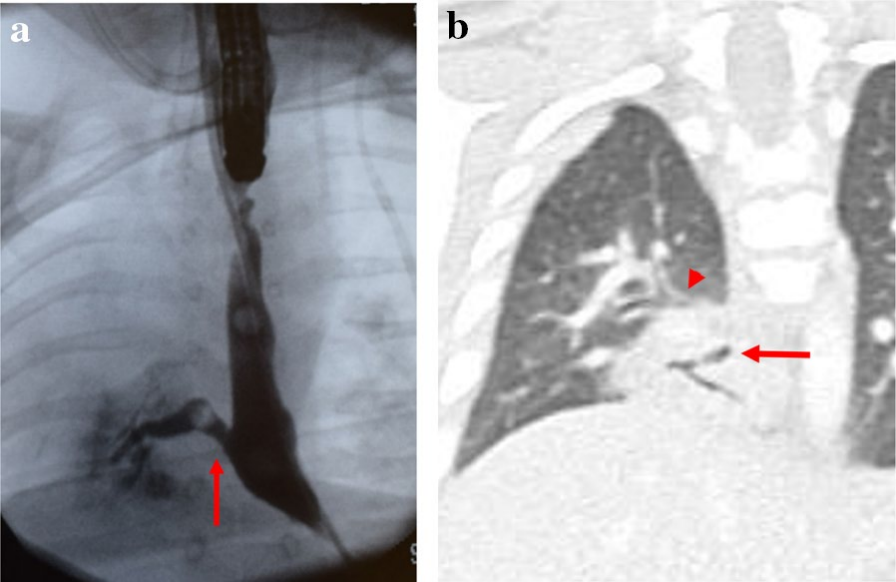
a An Esophagogram findings. The connection between the lower esophagus and right lower lung (arrow). b Computed tomography of the chest (coronal view). A connection between the esophagus and the right lower lung (arrow). Right main bronchus (arrowhead)
